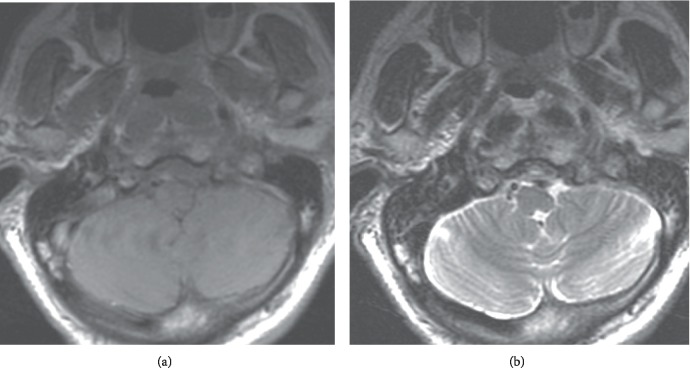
MRI assessment of the jugular foramen. Sagittal (a) and axial (b) MRI showed no signs of a space-occupying lesion or signal changes in the jugular foramen.
